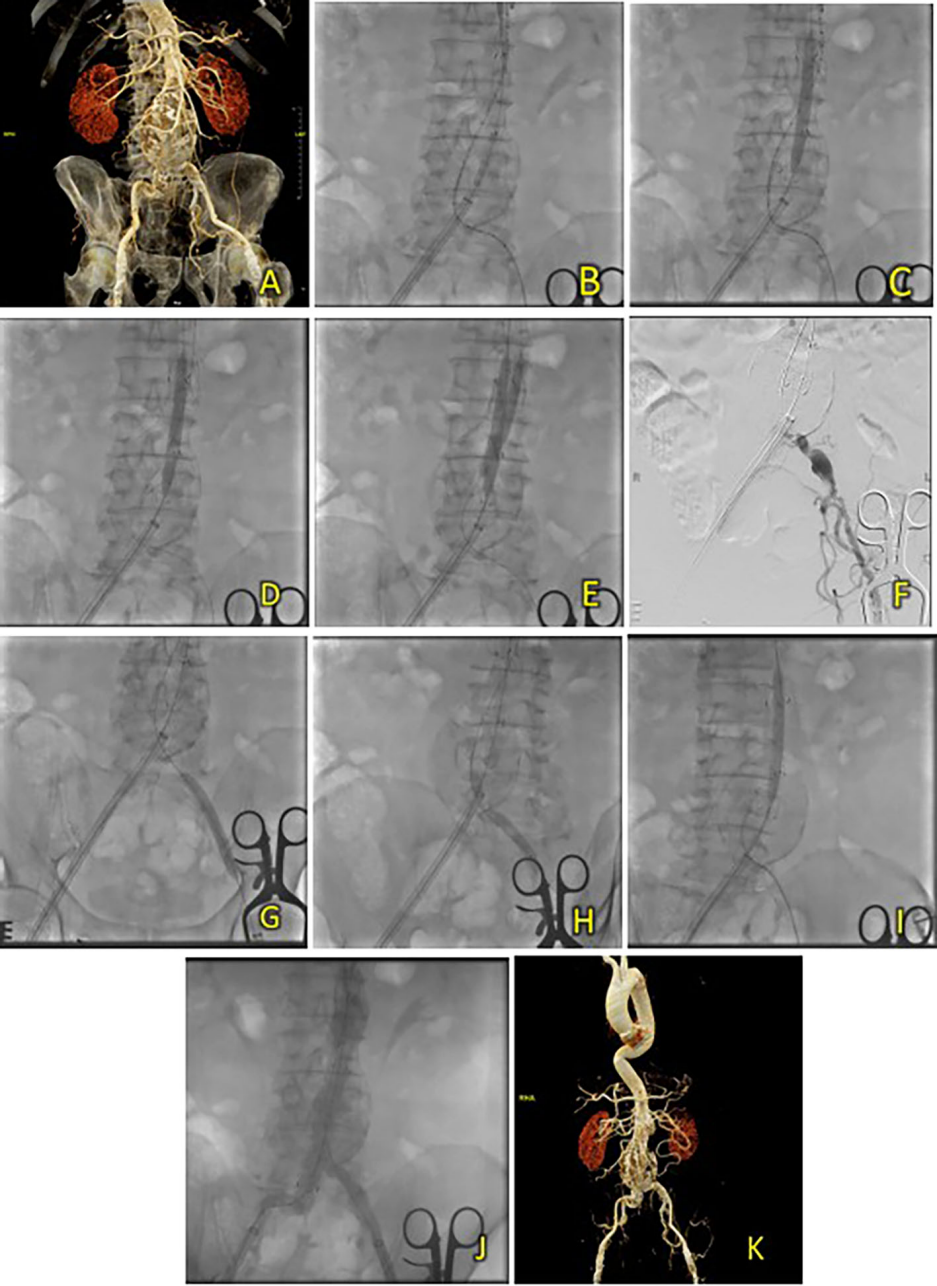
T&K B-EVAR on Patient 2. (A) Pre-procedure CT of the 2
